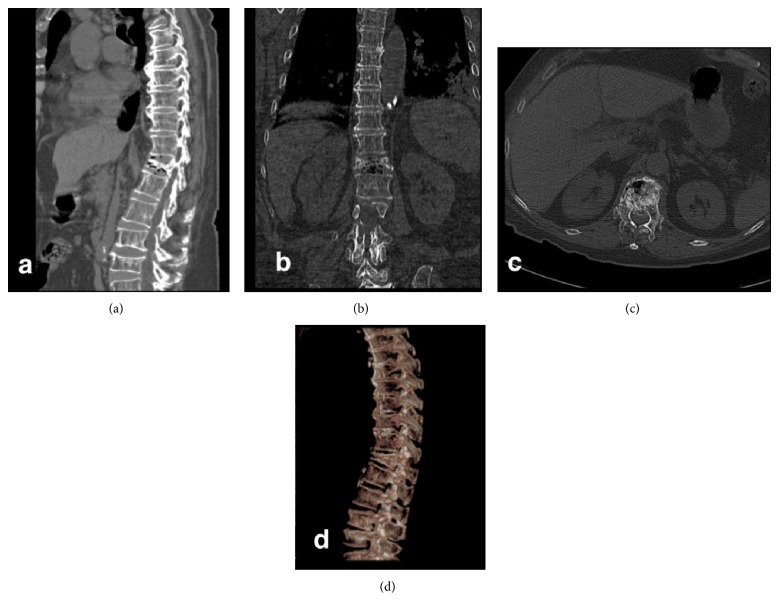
Computed tomography of thoracic spine in (a) sagittal, (b) coronal, and (c) axial projections revealed a T10 vertebral osteoporotic compression fracture and generalized spondylosis of thoracic and lumbar spine. Normal thoracic curvature was affected. (d) 3-dimensional images revealed in detail the wedge-shaped thoracic vertebrae and degenerative changes.
